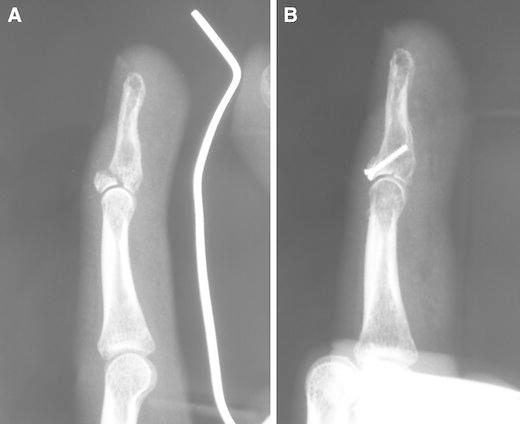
a Radiograph of a 38-year-old man with a type IIB mallet fracture. b Radiograph showing achievement of anatomical reduction
